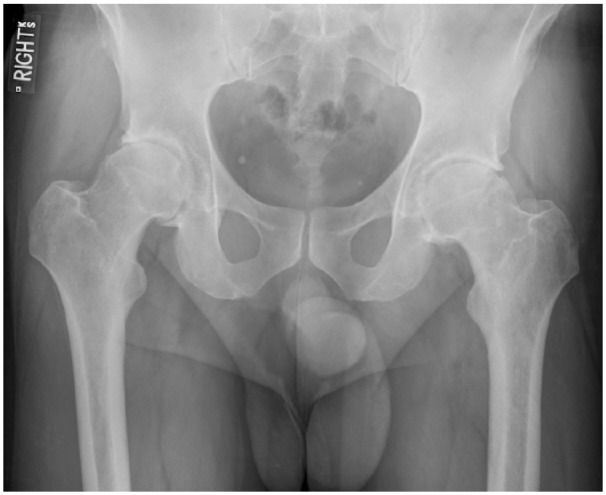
Pre-operative radiograph: pre-operative AP plain film radiograph
